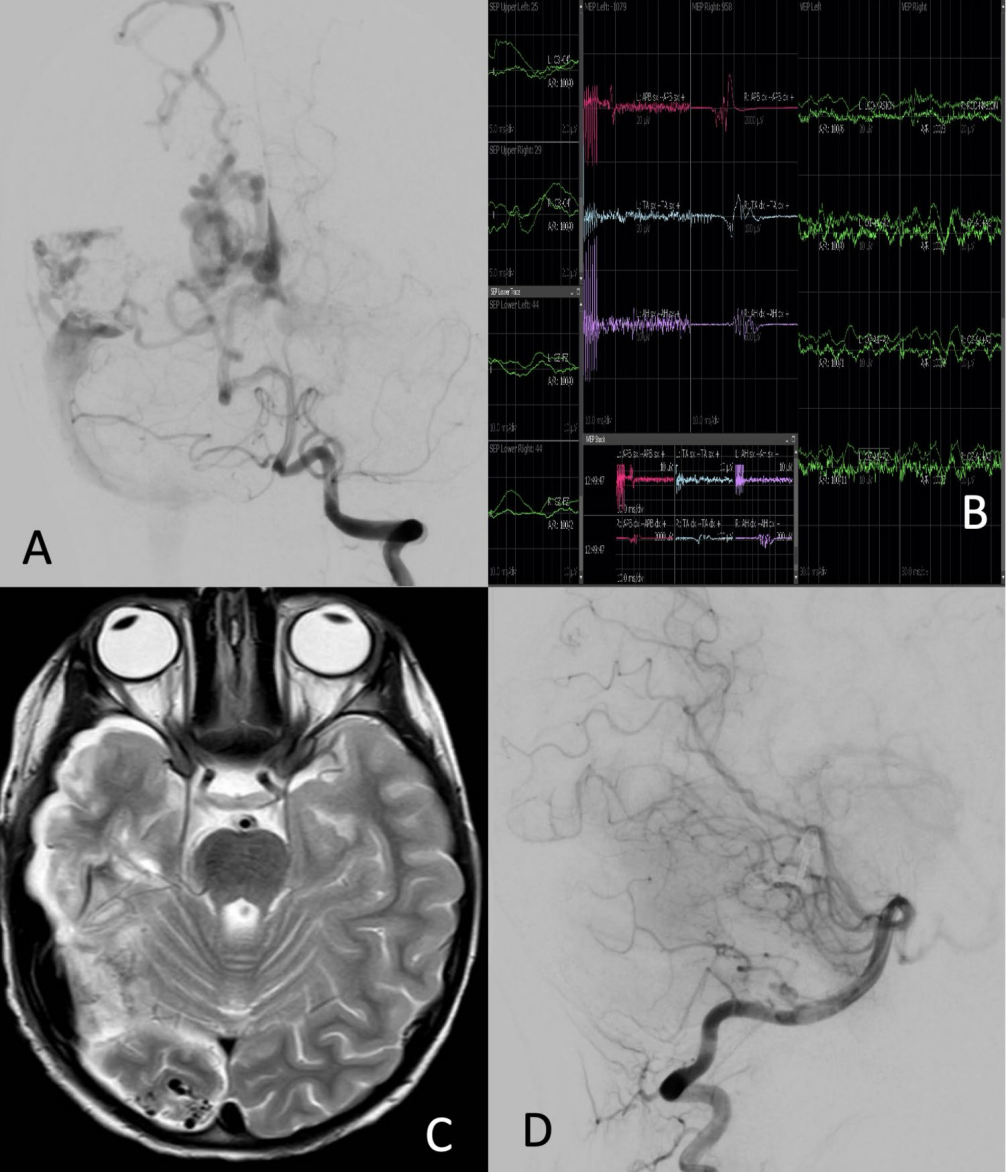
AVM case number 2. A Cerebral angiography documented the presence of two cerebral AVMs in the superficial occipital and temporal region. The occipital AVM was fed by an occipital branch of the right PCA and by a posterior temporo-occipital branch of the ipsilateral MCA. Two venous collectors were directed to the right internal cerebral vein and the superior sagittal sinus. The temporal AVM appeared dispersed into three small nidal compartments as a result of the previous surgery, fed by a temporal branch of the PCA and the posterior temporal branch of the MCA, with drainage to the transverse sinus and superior sagittal sinus. B MEP, SSEP, and VEP monitoring: before surgery, left SSEPs and MEPs were absent, and left VEPs were also reduced. At the end of the procedure, potentials were unchanged from baseline. C Brain MRI before the last surgery for resection of the occipital AVM. D Post-operative angiography at 3 years showing no residual malformation
